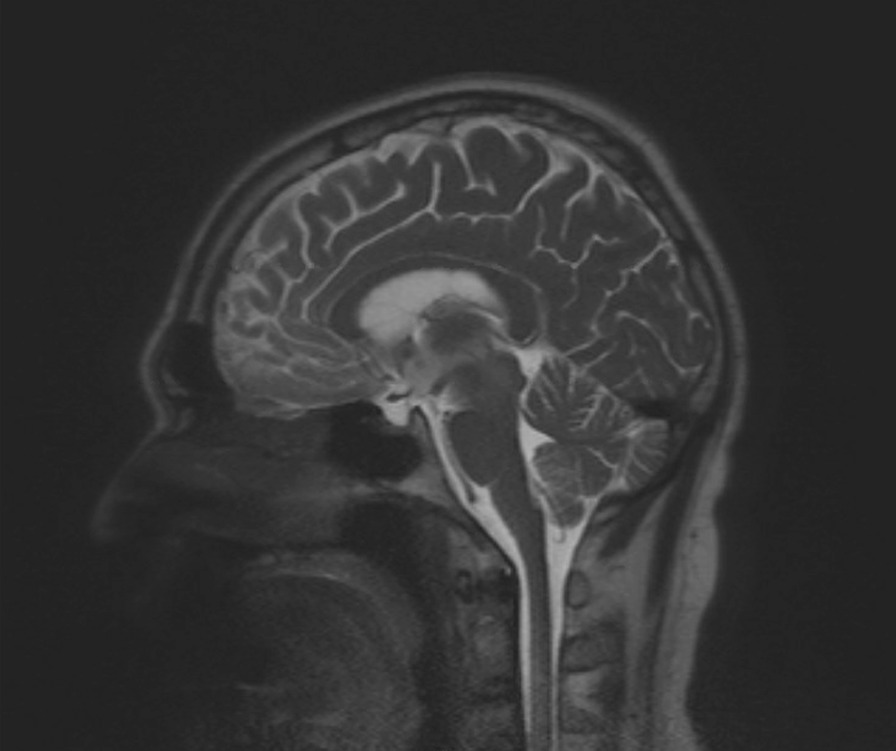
Magnetic resonance imaging of pituitary and brain showing symmetric T2/fluid-attenuated inversion recovery (FLAIR) hyperintensity in the mesial temporal lobes, hippocampi, hypothalamus, mamillary bodies, and midbrain including periaqueductal gray matter. Sella turcica and pituitary gland are normal in size
